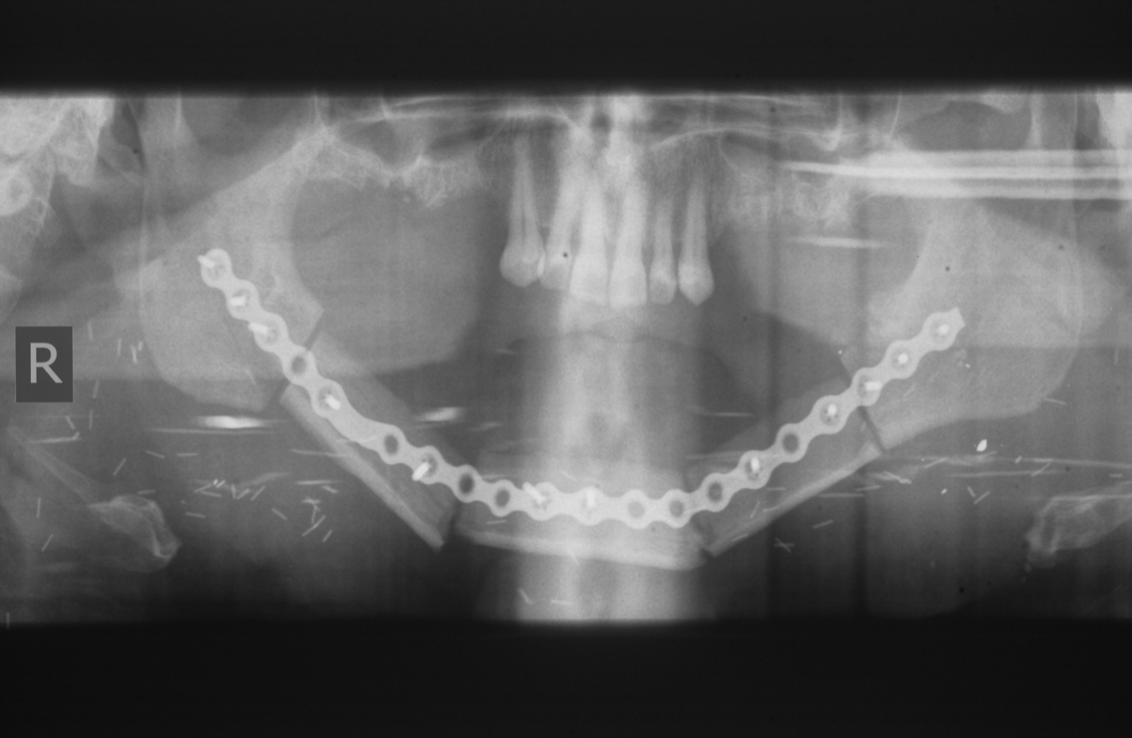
Fibula osteocutaneous flap for reconstruction of the mandible. Postoperative X-ray 3 months after transplantation
